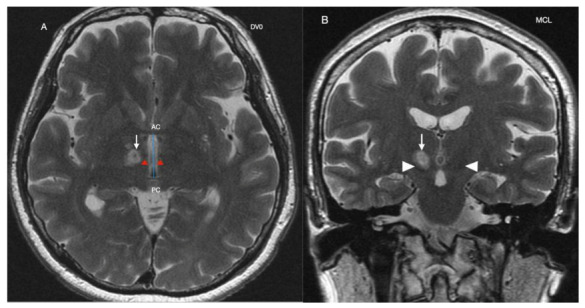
Cranial MRI of Case 2 at day 1 post-pallidothalamic tractotomy. (A): Axial cuts at DV0 showing the right pallidothalamic tract target lesion (white arrow) on postoperative day 1. The intercommissural line (blue line) is the distance between the anterior commissure (AC) and the posterior commissure (PC). Red arrows point to the mamillo-thalamic tract. (B): Sagittal scan at midcommissural line (MCL). White arrow is target lesion. Arrow heads are the subthalamic nuclei.
